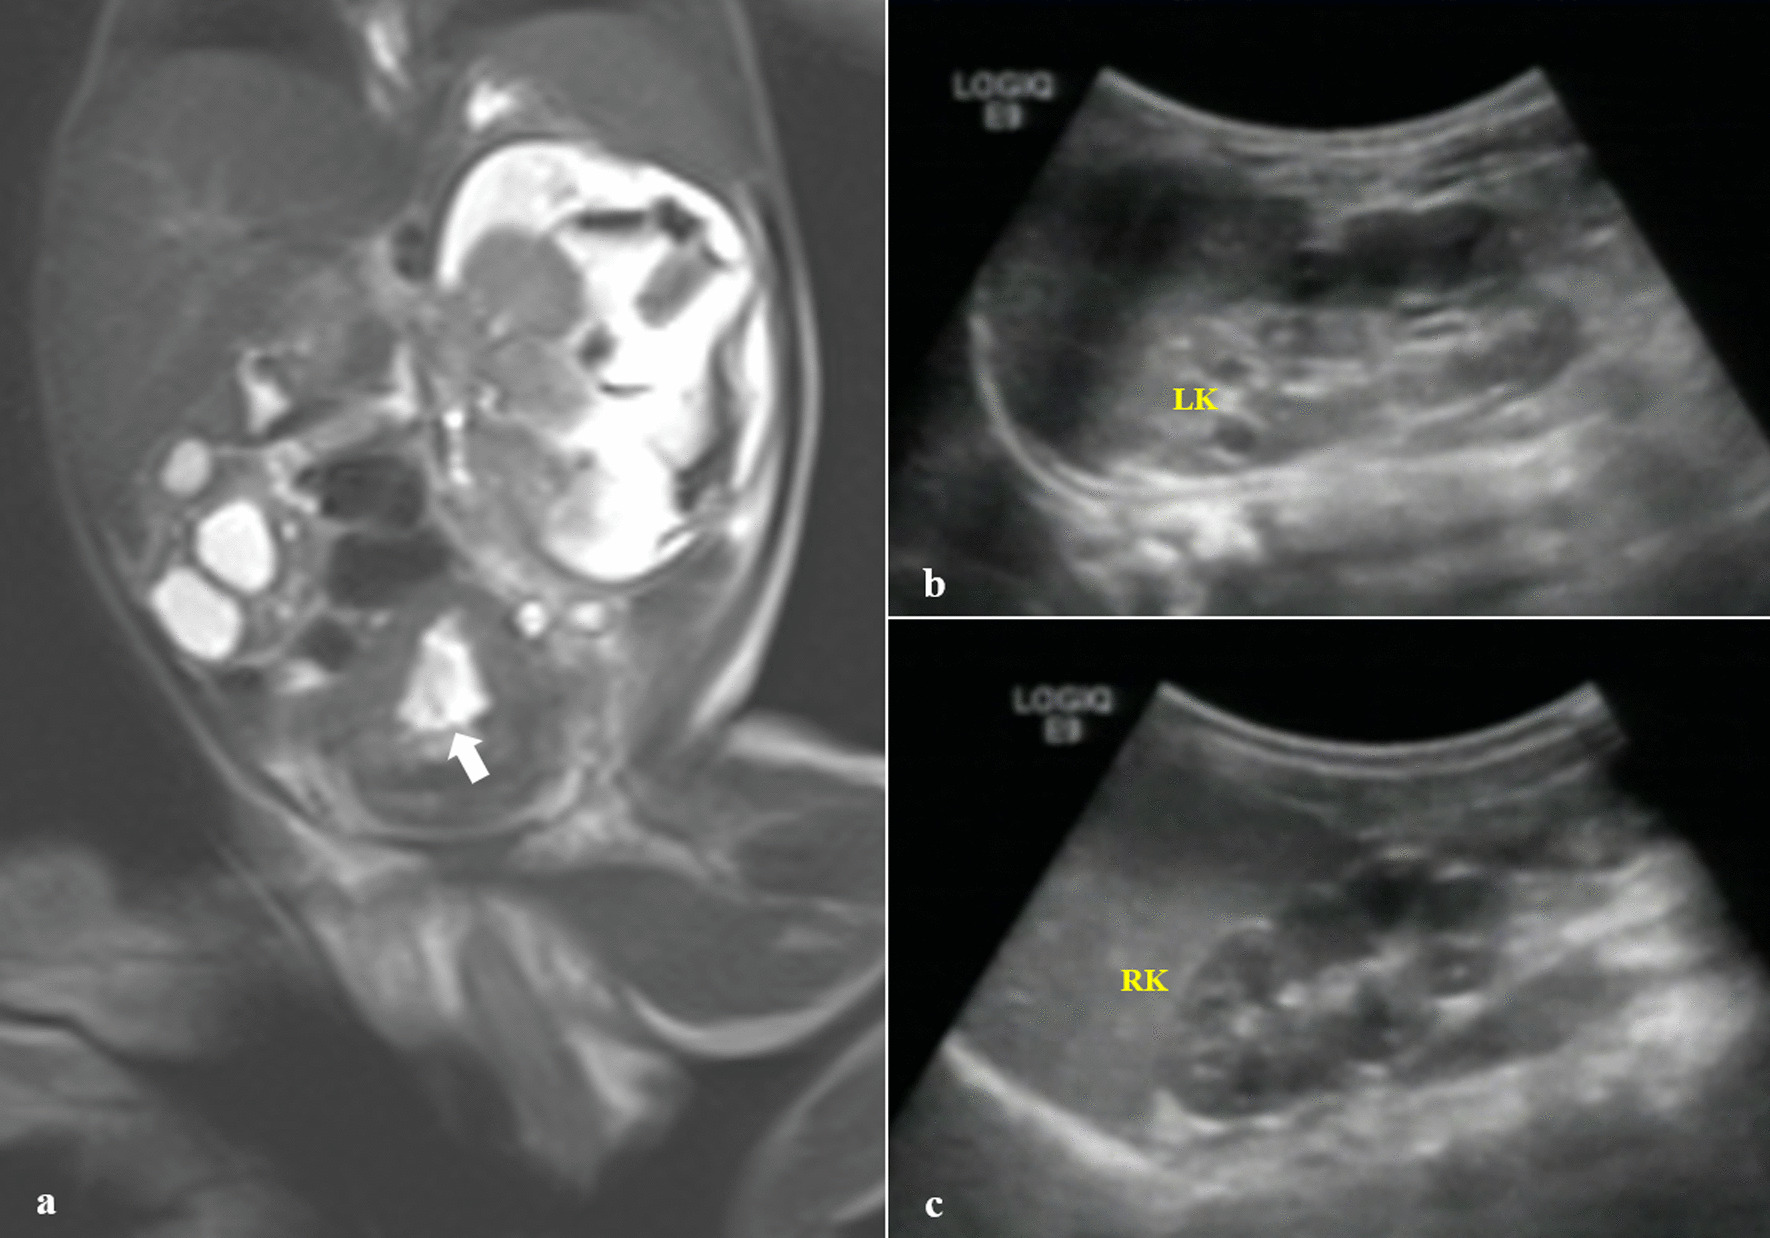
a A T1-weighted coronal view revealed a bladder wall irregularity and thickening. b, c Ultrasound images of both kidneys at 8 months. Thick arrow showing bladder
